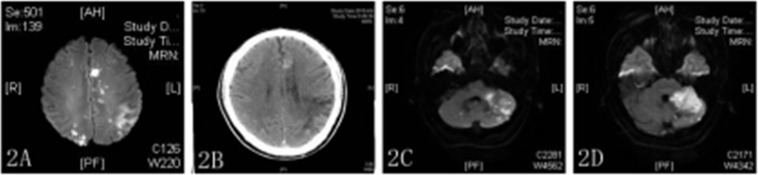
(A,C,D) DWI showed multiple high-density areas in the brain (A) and high-density shadows in bilateral cerebellar hemispheres (C,D). (B) CT image showing a new high-density shadow.
